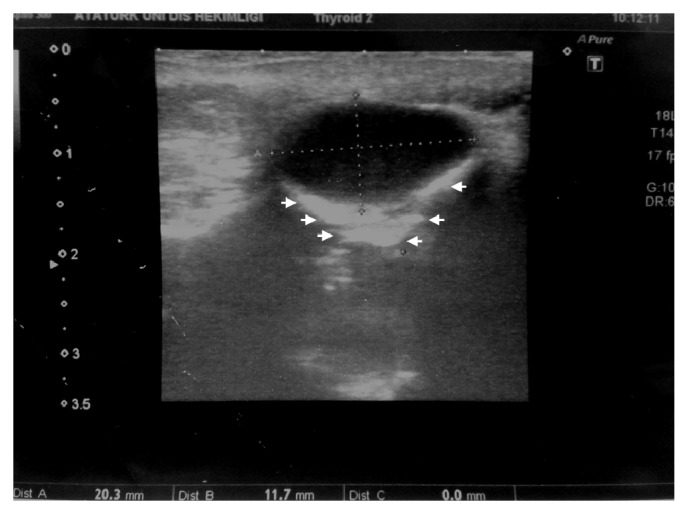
Ultrasonography shows well-defined anechoic cystic lesion about 2 cm diameter with posterior acoustic enhancement (arrows).
